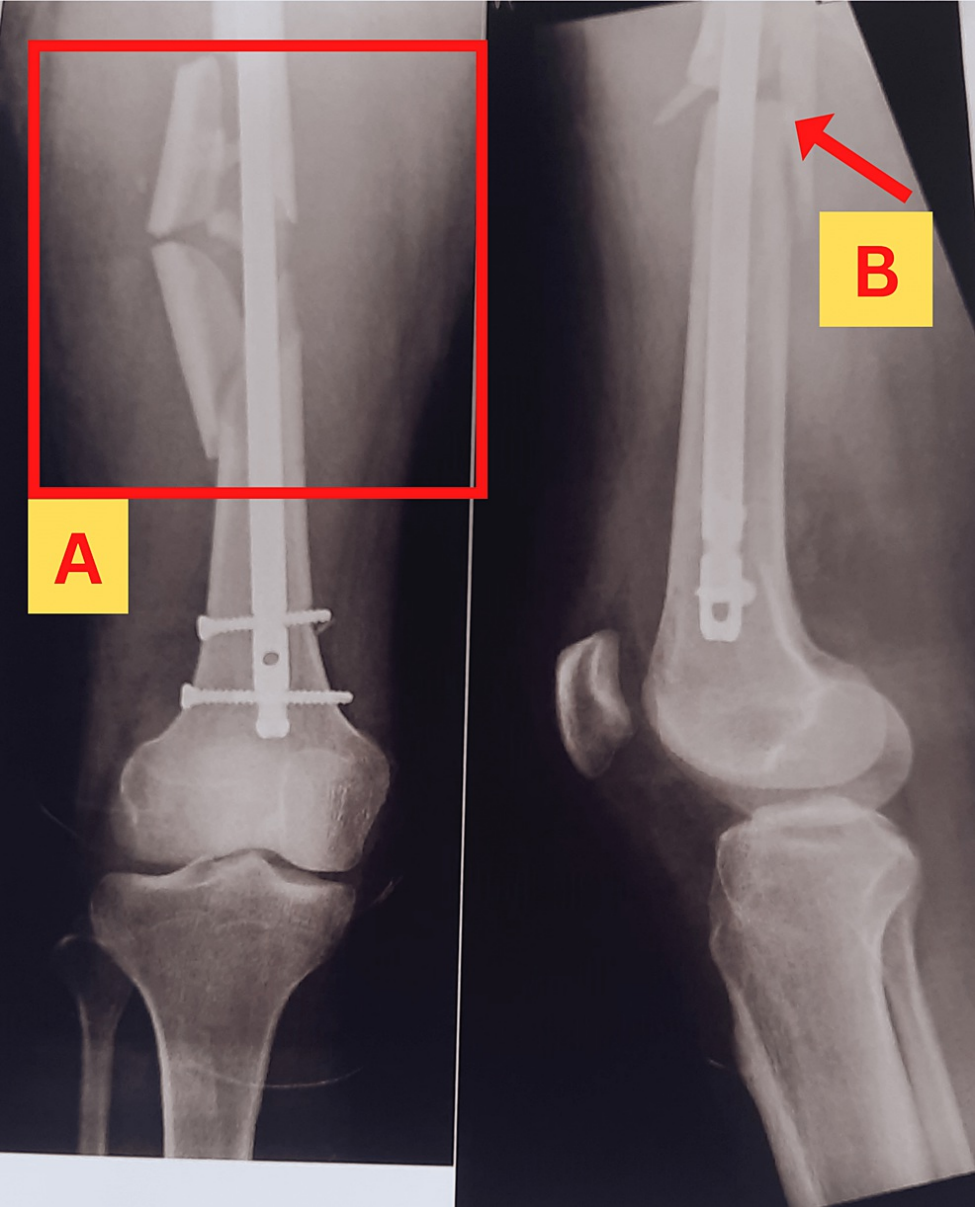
X-ray images of the shaft of the femur with PFN fixation (A) AP (anteroposterior) view; (B) lateral view Square A shows the comminuted femoral shaft fracture in the AP view, while arrow B points at the fractured femoral shaft in the lateral view. PFN: Proximal femoral nailing.
